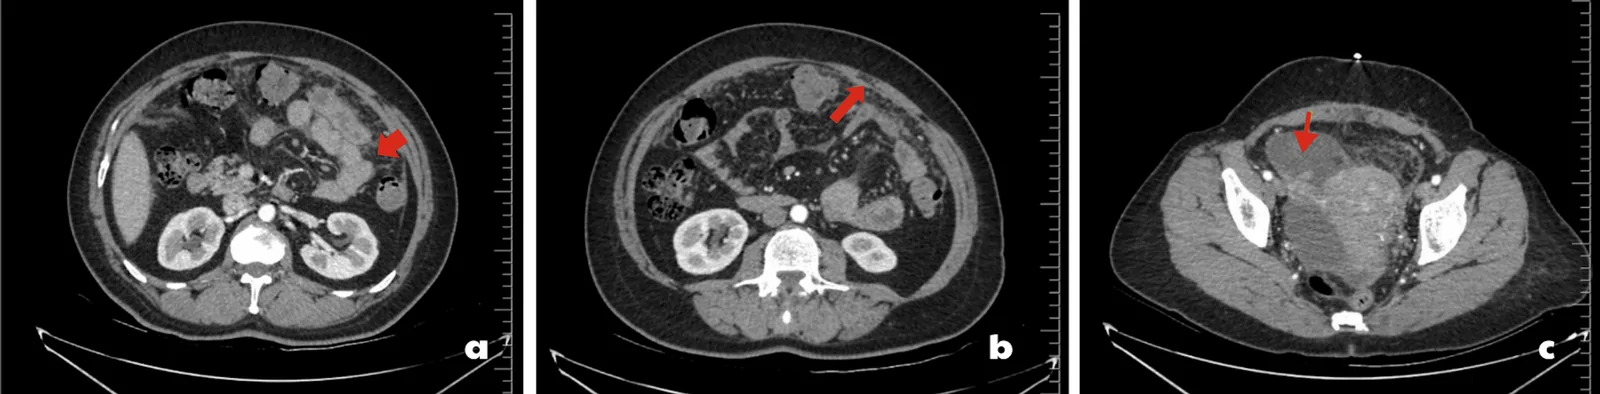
Preoperative CT images of the patient The patient's CT abdominal examination revealed heterogeneity in the omental fatty tissue (a). Peritoneal thickening (b). Free intra-abdominal fluid (c). The findings primarily suggest peritonitis. Differential diagnosis includes peritonitis carcinomatosis.
